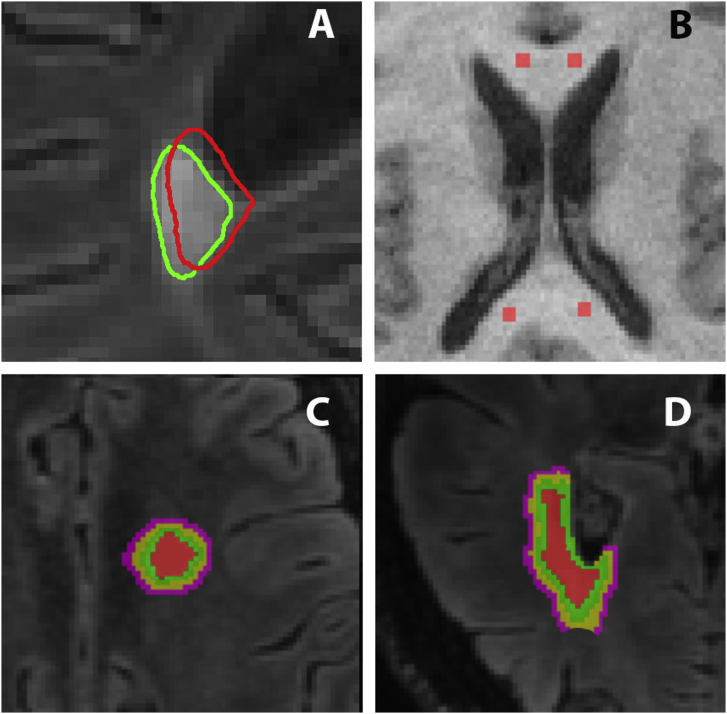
Lesion and NAWM ROIs. A. Examples of lesion position adjustment (lesion shifting outwards). Red contour: baseline lesion, green contour: follow-up lesion displayed on follow-up T2 FLAIR image. B. Example of 4 ROIs used for NAWM. C. Segmentation of lesional and perilesional white matter displayed on baseline T2 FLAIR image. Red colour represents lesion core, green colour represents lesion “rim” and PLWM shells are yellow and magenta. D. Example of removing CSF contamination from PLWM displayed on baseline T2 FLAIR image. (For interpretation of the references to colour in this figure legend, the reader is referred to the web version of this article.)
